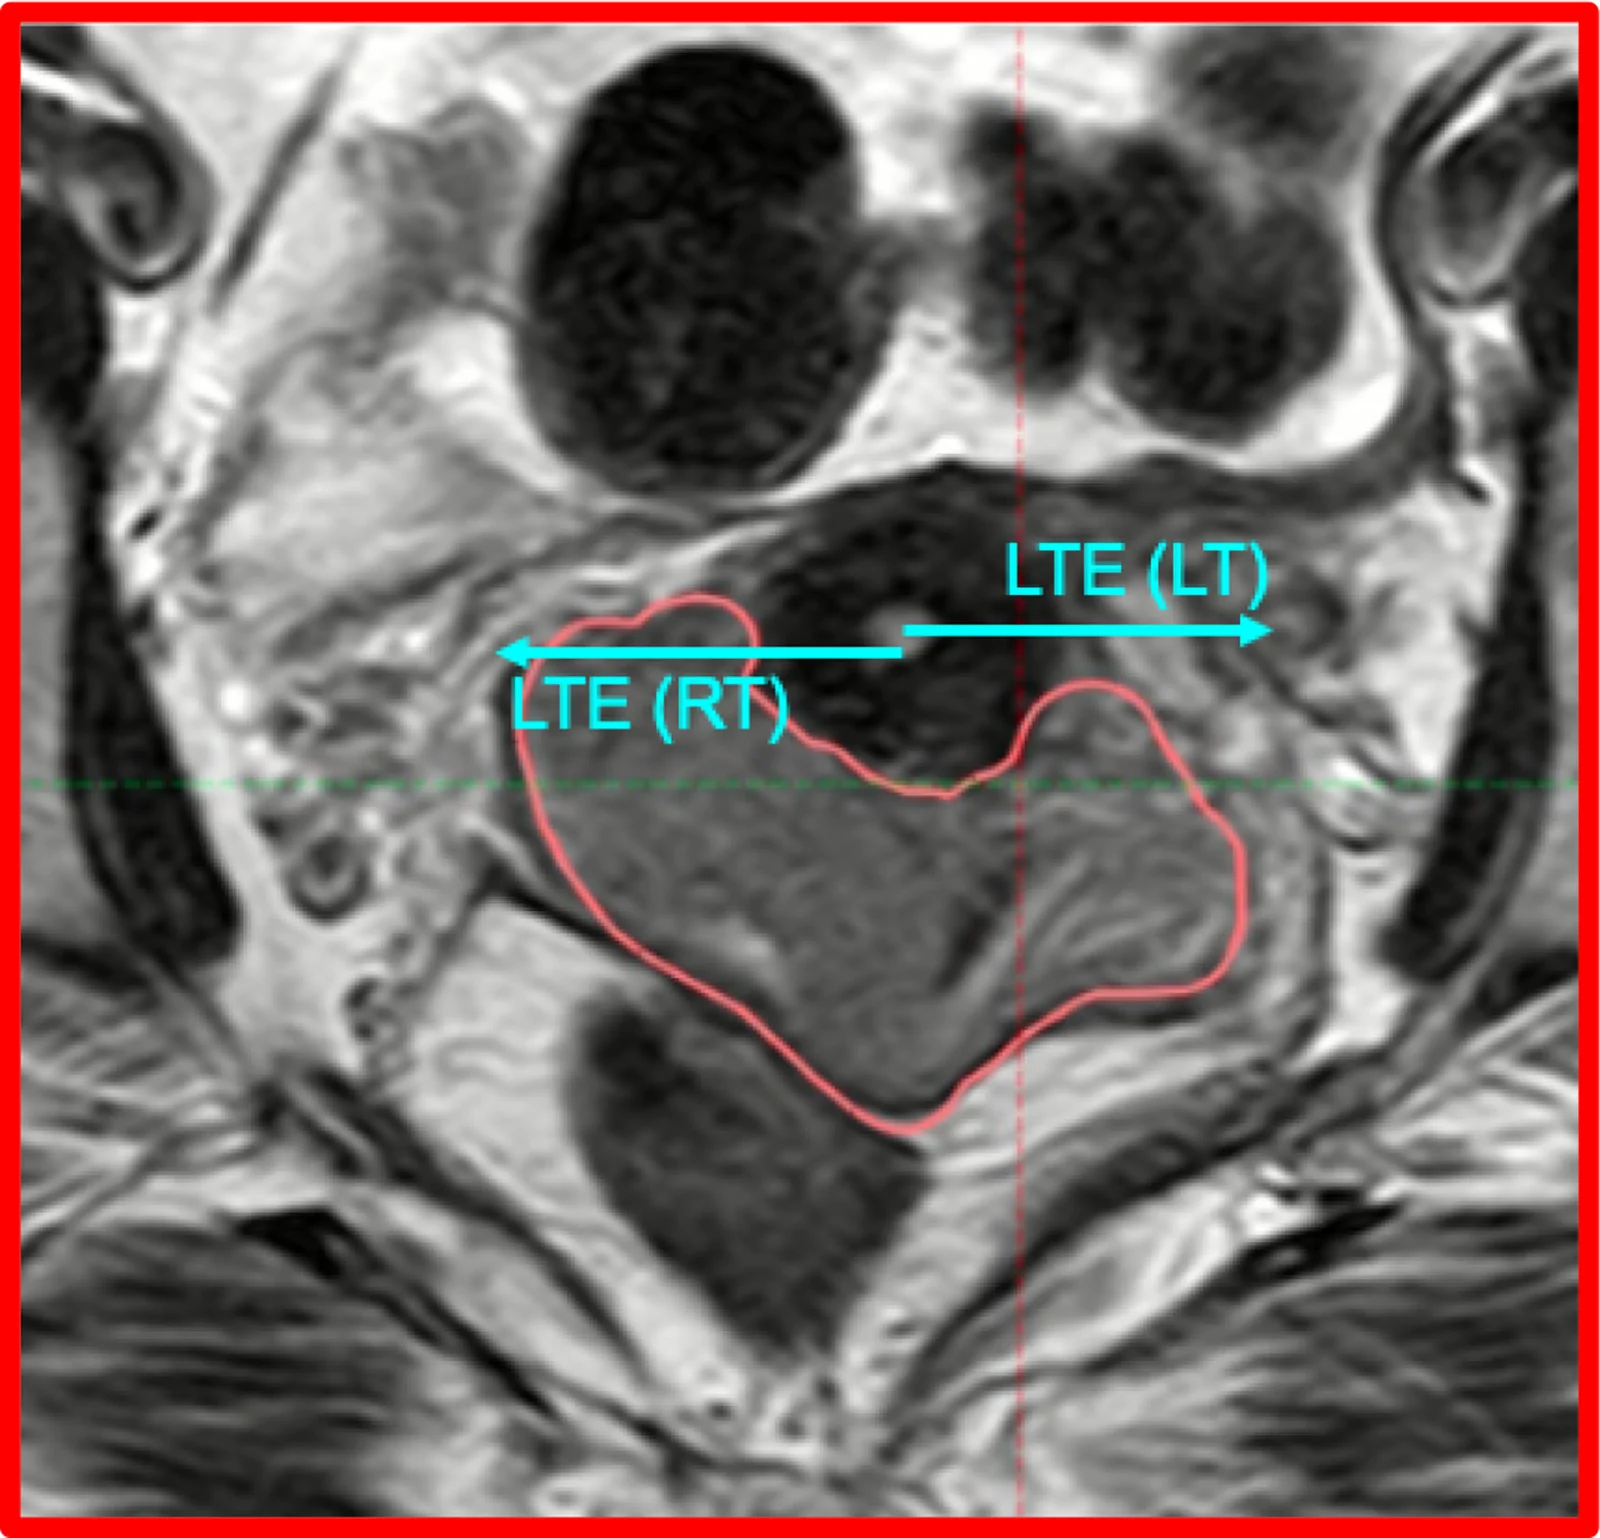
a. Figure depicting GTV_T2 in red outline and GTV_T2/DWI in cyan outline and the overlap volume in dotted blue outline. (For interpretation of the references to colour in this figure legend, the reader is referred to the web version of this article.) b. Figure depicting the measures of Lateral Tumor Extent on axial T2 weighted MRI on right, LTE (RT) and left side, LTE (LT).
